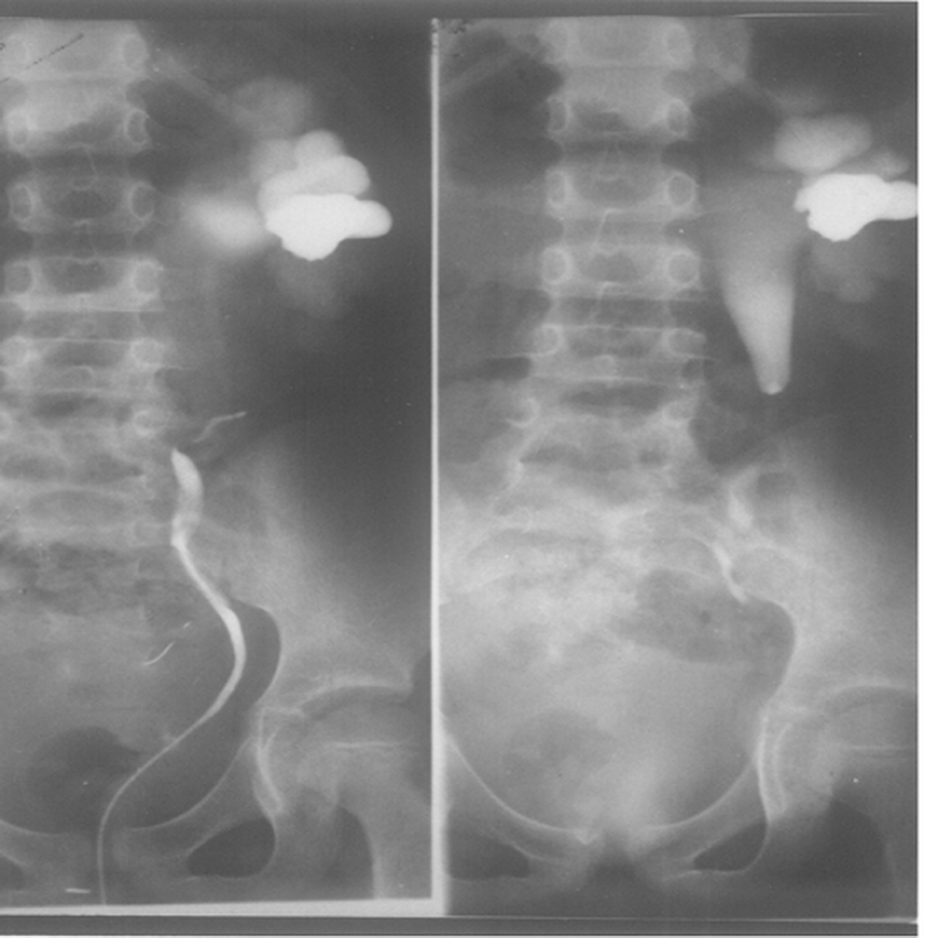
Ureter stricture seen under X-ray retrograde urethrography and descending urography. The results from X-ray retrograde urethrography and descending urography of the patient described in Figure 2.
